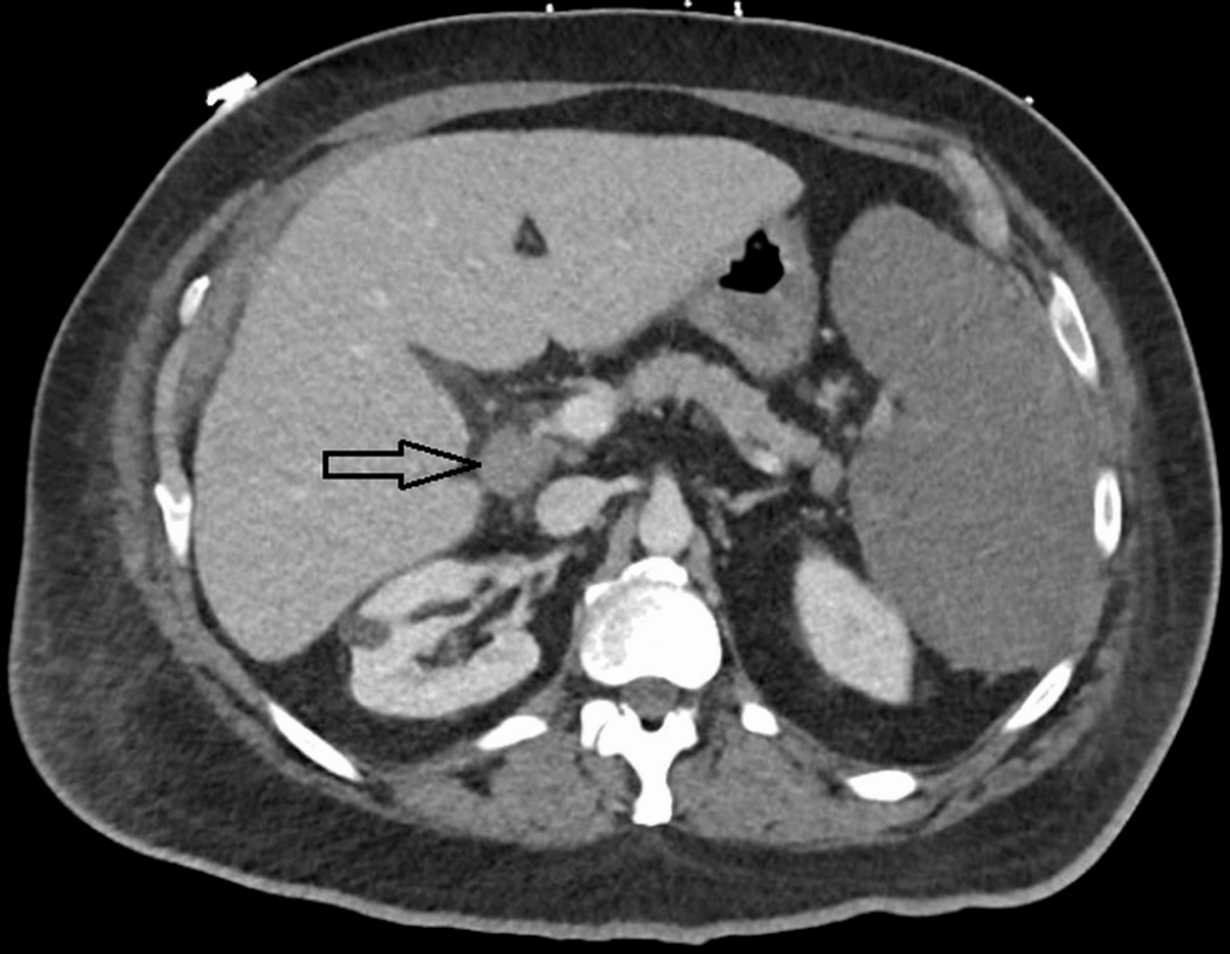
Computed tomography (CT) of the abdomen demonstrating hepatosplenomegaly and marked porta hepatis lymphadenopathy (arrow).
